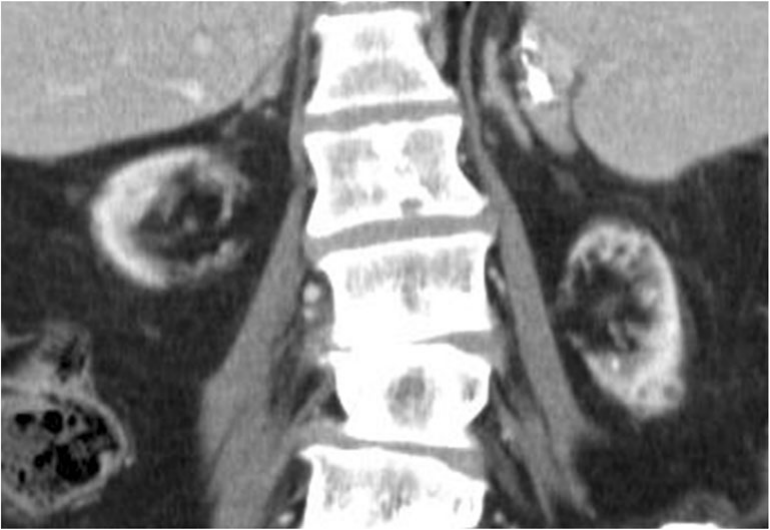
Chronic renal failure in a 76-year-old female. CT image of kidneys reveals a short length and diffuse reduction of renal cortical thickness
